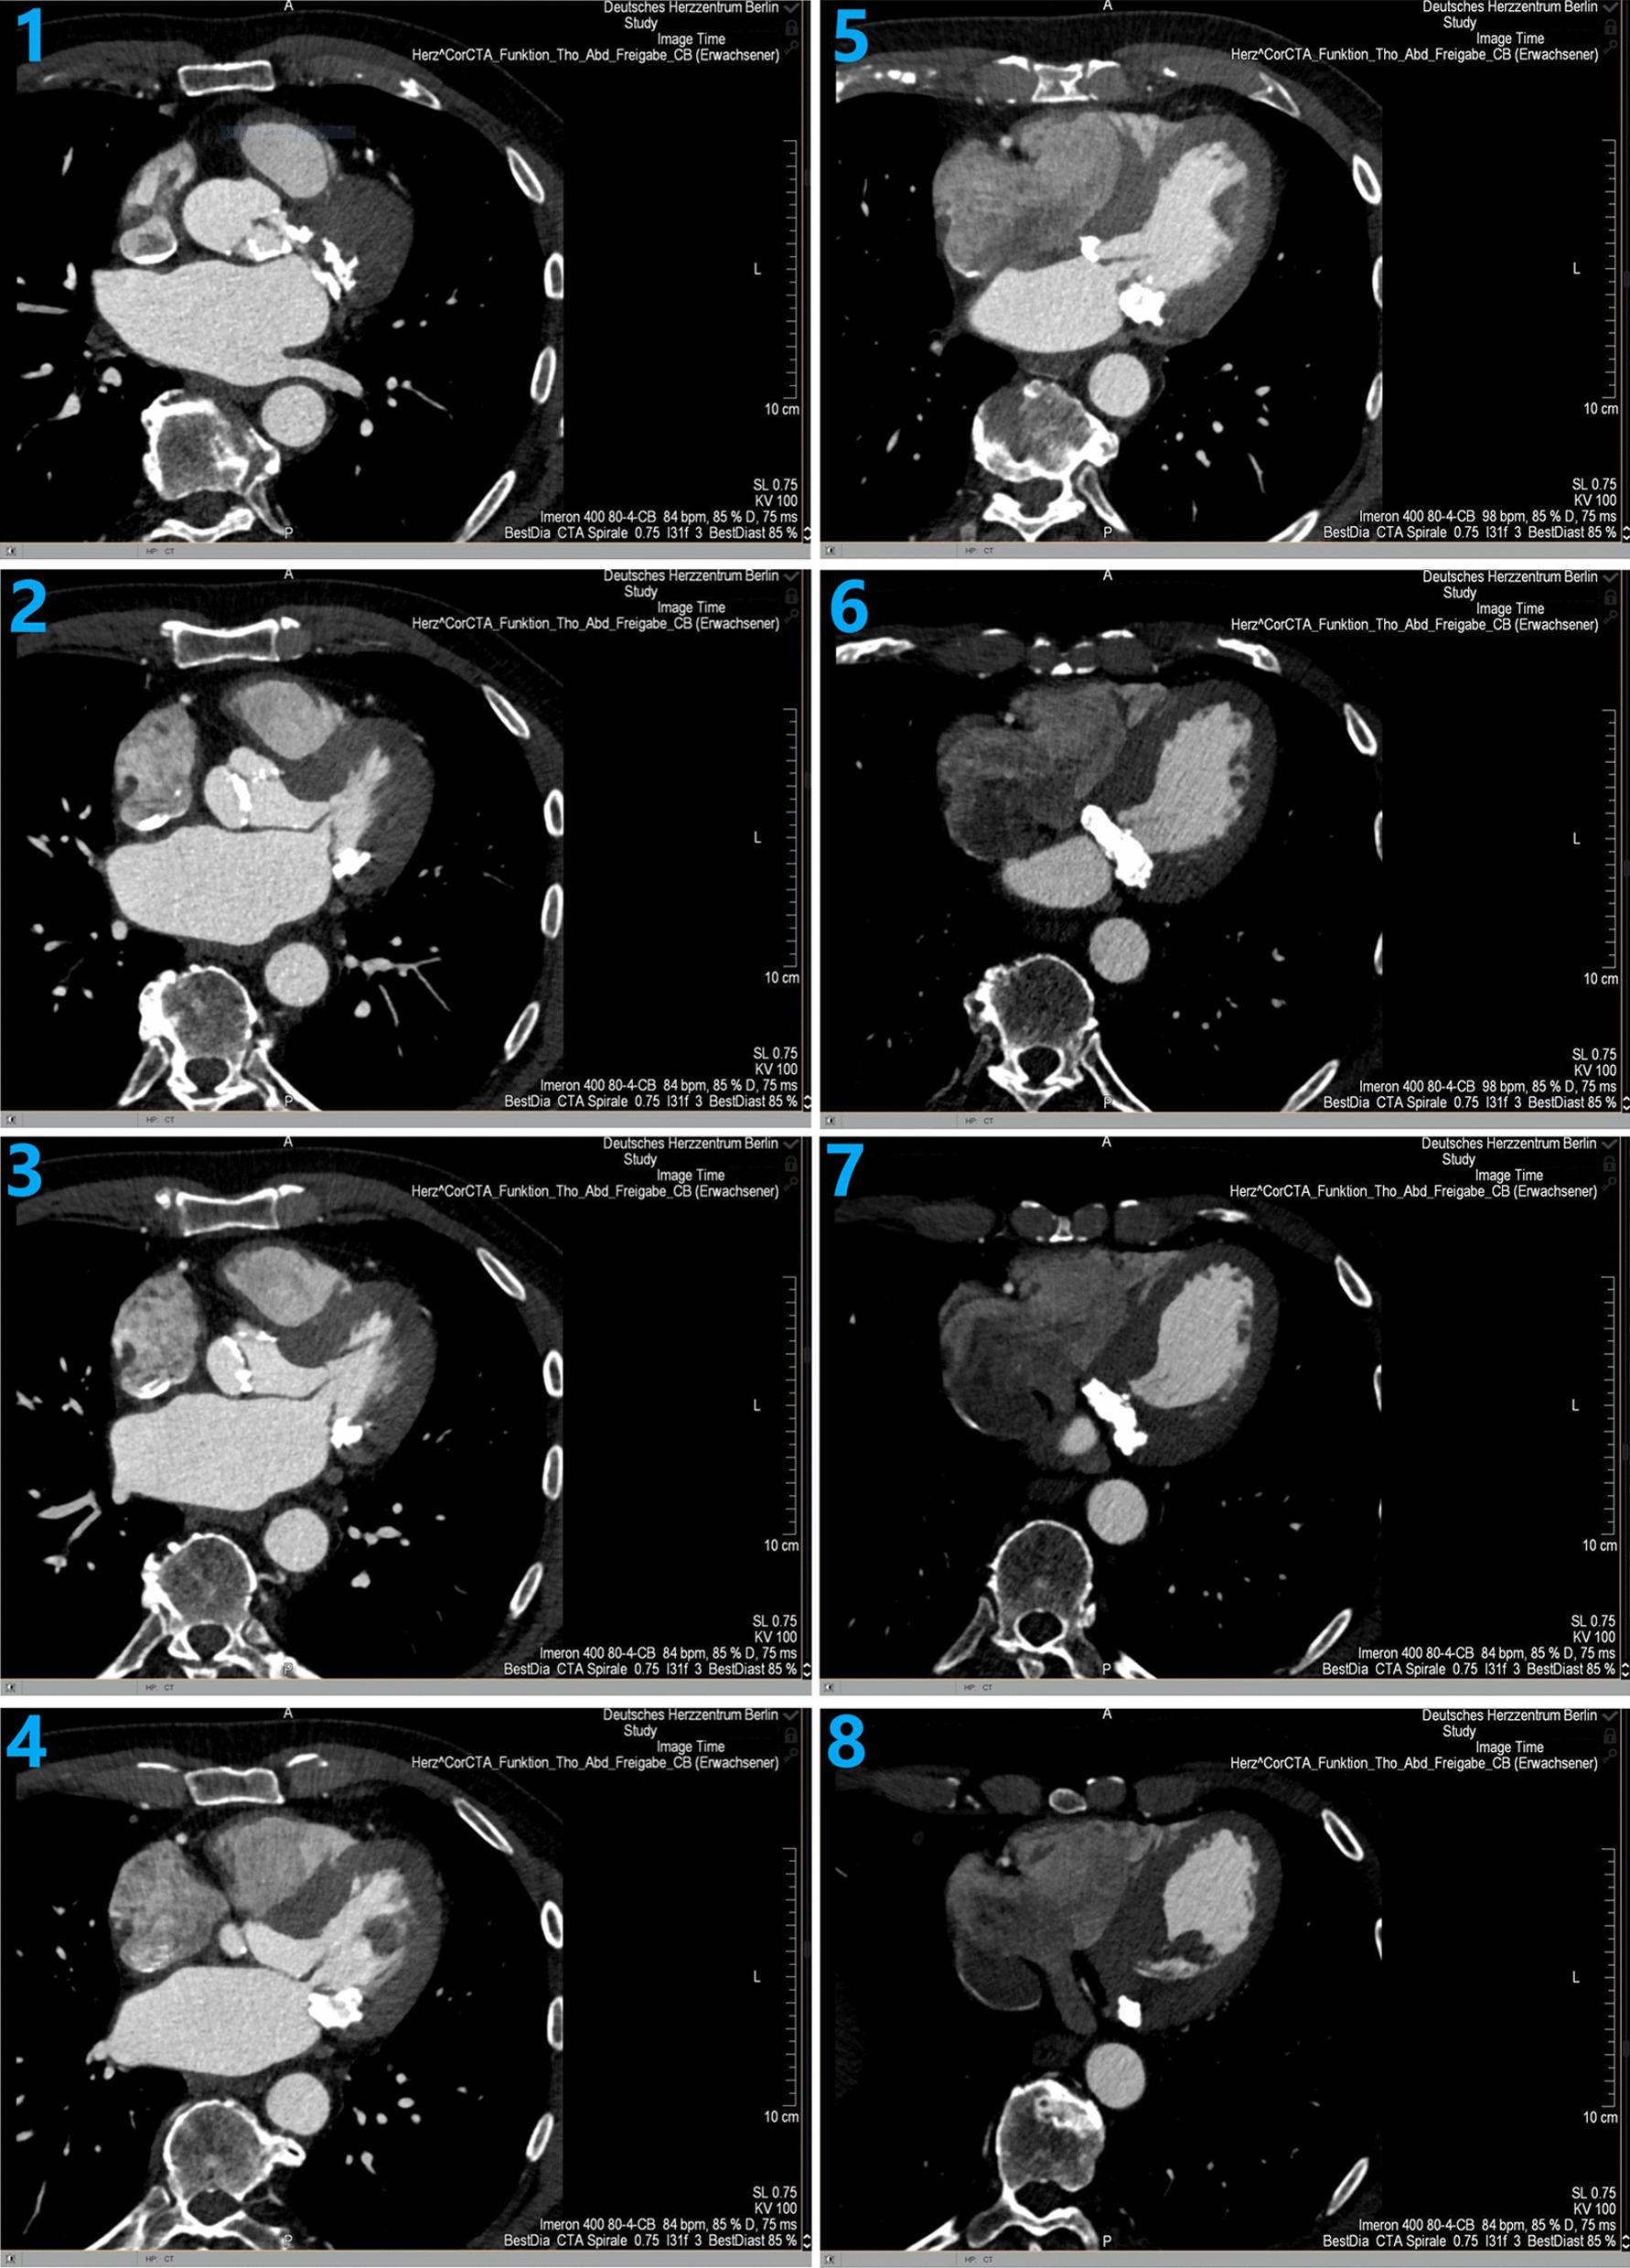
Preoperative CT scan showing massive calcification of the mitral valve and the mitral annulus with involvement of the left atrium, the left ventricle and the left outflow tract
